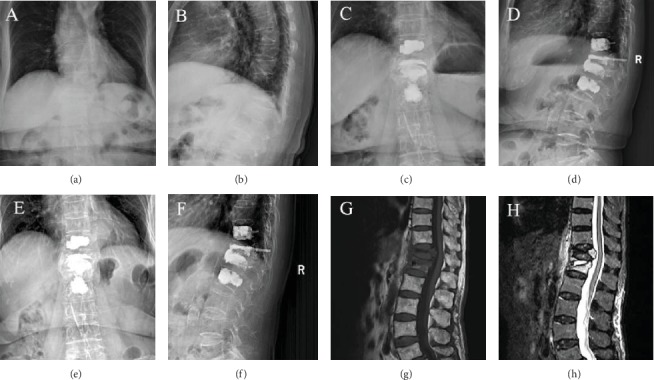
Preoperative X-ray of a 76-year-old woman with OVCF of the T12 and L1 vertebral bodies (a, b). MRI (T1-weighted and STIR-weighted images) visualized each fractured vertebral body (g, h). The BMD values were -3.9 (T12) and -3.4 (L1). The patient underwent PKP from T12 to L1, and T11 and L2 were treated with prophylactic PKP. X-rays were obtained after PKP (c, d). Twelve months after PKP, no evidence of new VCFs was observed (e, f).
